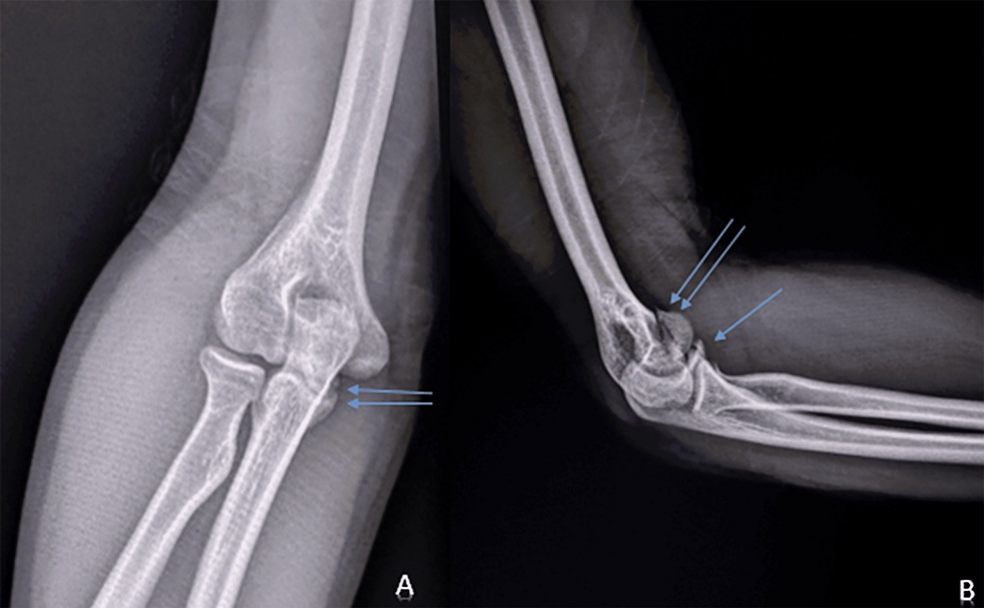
Standard radiograph in anteroposterior (A) and lateral (B) views showing a fracture of the humeral trochlea (two blue arrows) and avulsion of the coronoid process (one blue arrow)
